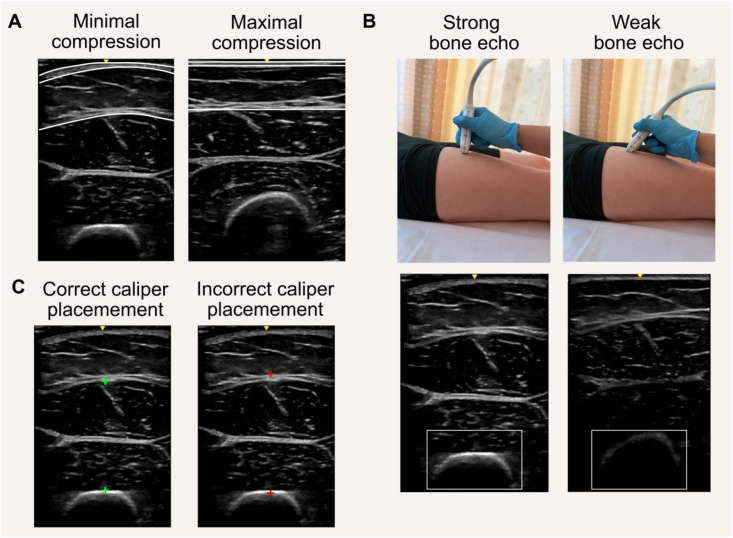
Practical considerations for body composition assessment using ultrasound. (A) A reliable approach to confirm that an ultrasound image was captured with minimal compression is by identifying a visible skin arch in the image. (B) Changes in probe tilt can be identified by the bone echo: correct/neutral probe tilt produces strong bone echo; incorrect tilted/inclined probe produces weak bone echo. (C) Correct placement of calipers within the ultrasound image for accurate measurements of the quadriceps (rectus femoris + vastus intermedius) muscle thickness.
